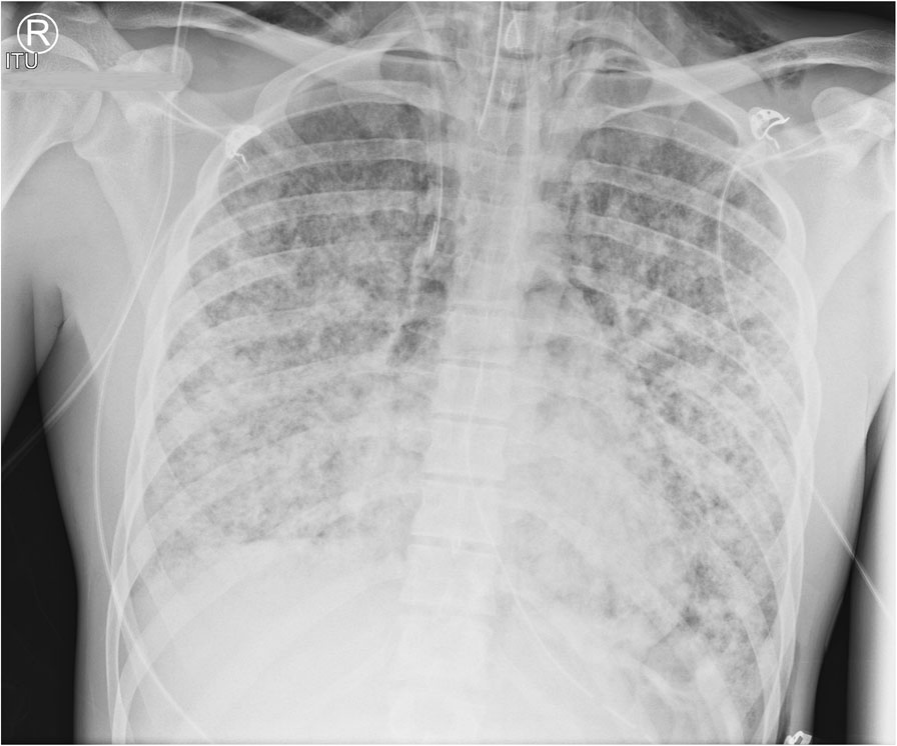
Chest X-ray of patient on admission to local hospital post-intubation
